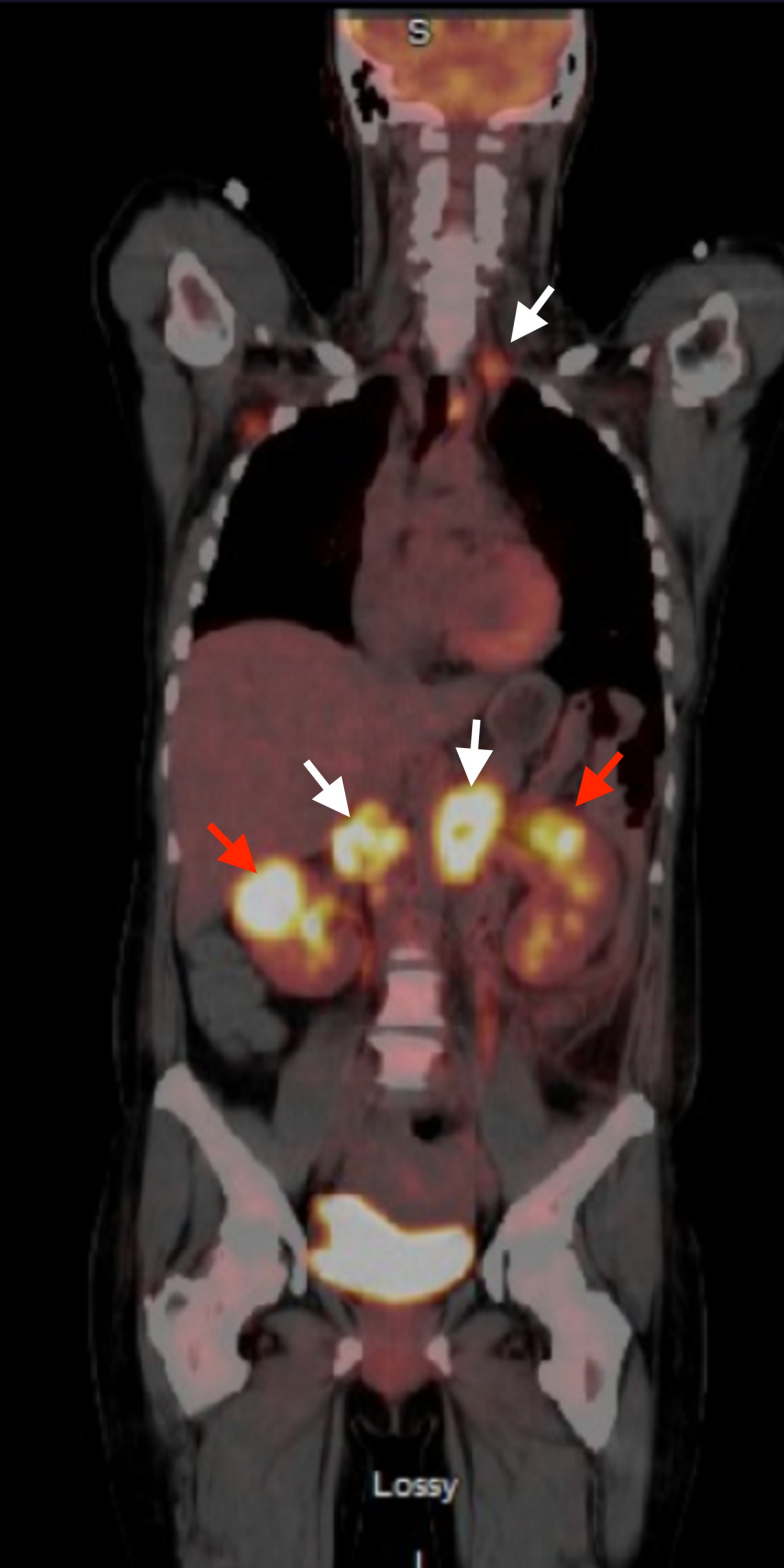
Positron emission tomography/computed tomography (PET/CT) on presentation Left supraclavicular and retroperitoneal lymphadenopathy (white arrows) with hypermetabolic bilateral renal masses (red arrows).
